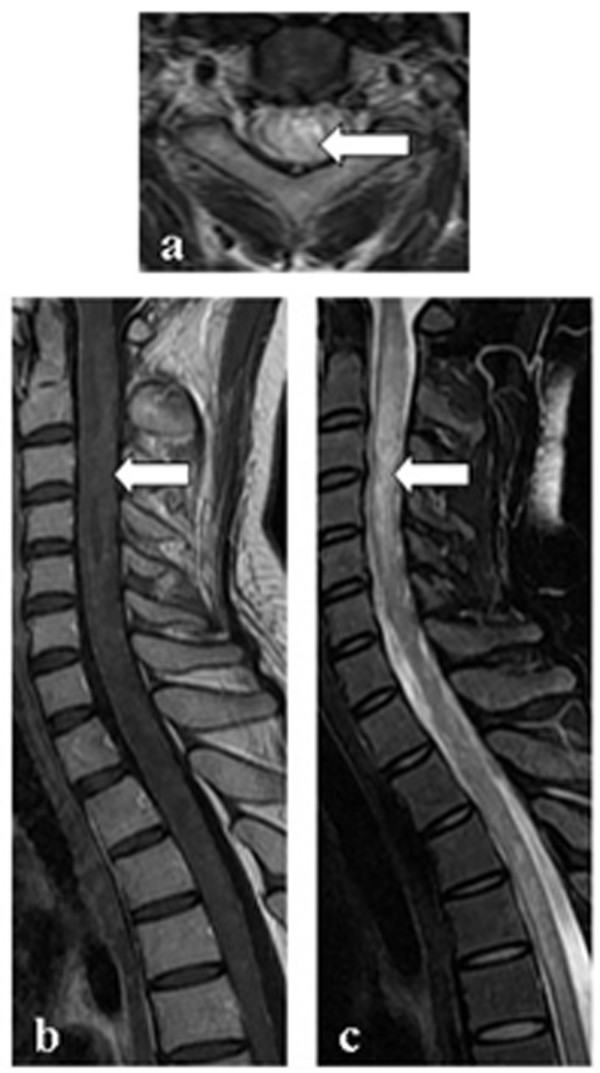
Spinal MRI of the NMO patient with active PTB. a: axial T2-weighted image showing a cervical lesion predominantly involving the central cord; b: T1-weighted contrast image revealing slight enhancement of the lesion; c: T2-weighted image showing a longitudinal extensive spinal cord lesion.
